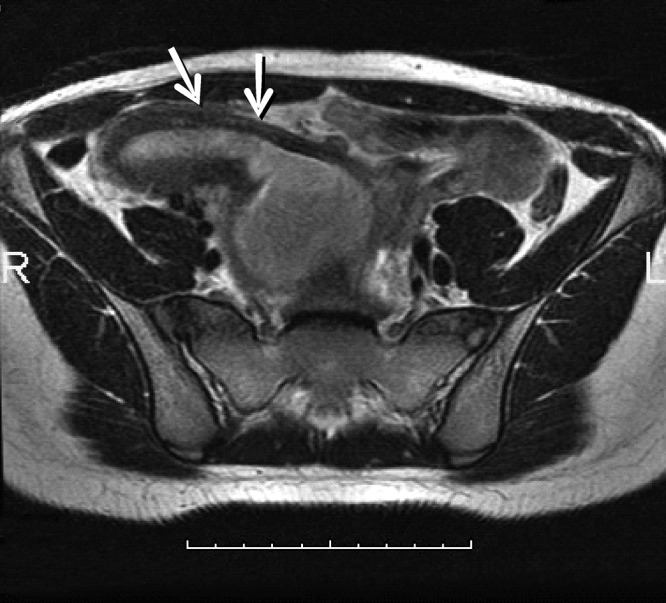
Figure 2:MRI showing dilated (right) and normal cavity (left) images of the uterus, fundus and cervix (arrows)
